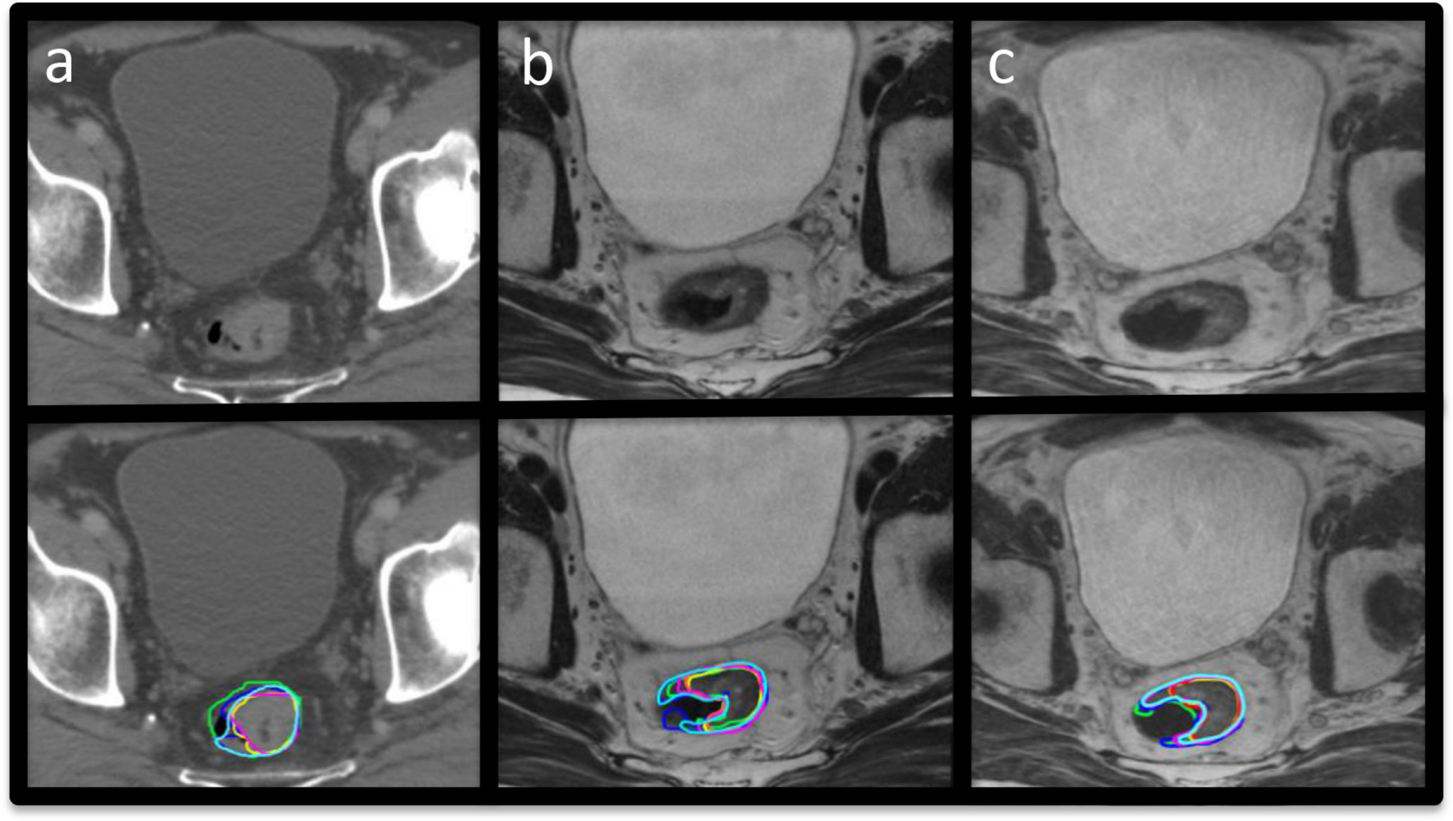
Example images with and without observer GTVp contours at week 0. (a) CT, (b) 2D-MRI and (c) 3D-MRI. GTVp, gross tumour volume.
